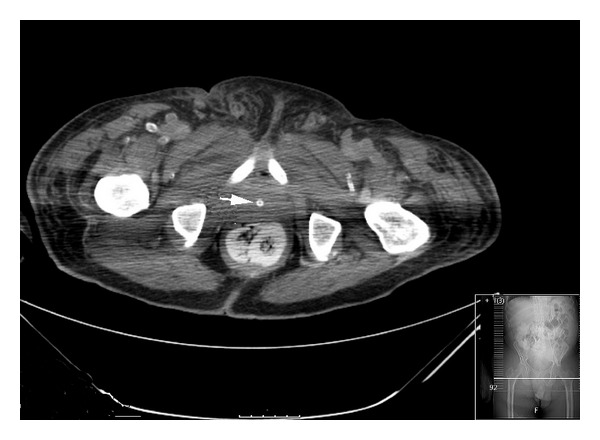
Patient 2: CT showing (arrow) the urethral catheter lodged in the prostatic urethra inducing obstruction to urinary flow.
